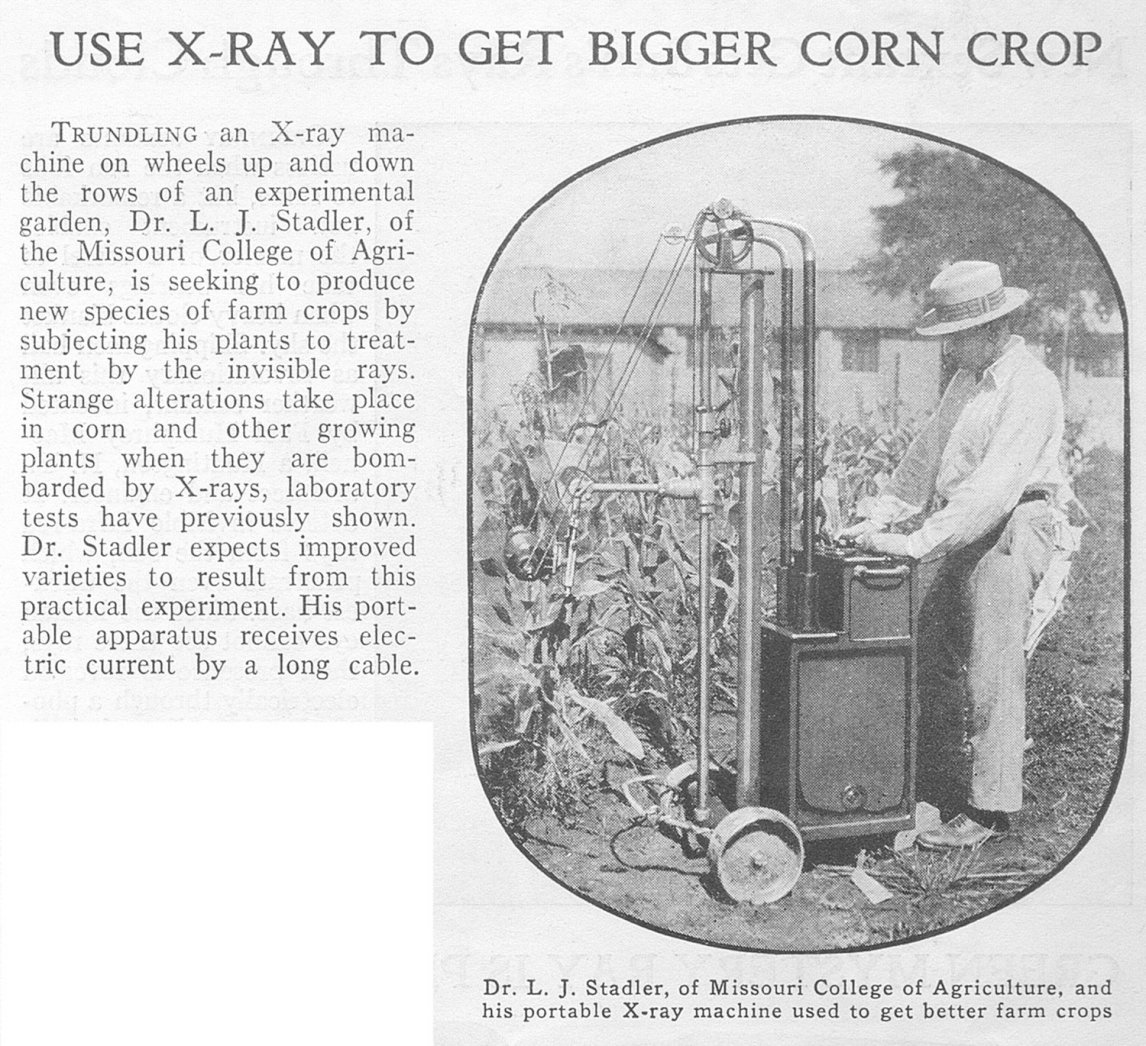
The geneticist Lewis Stadler X-rays corn in hopes of inducing mutations. From *Popular Science Monthly*, January 1932, 47.
